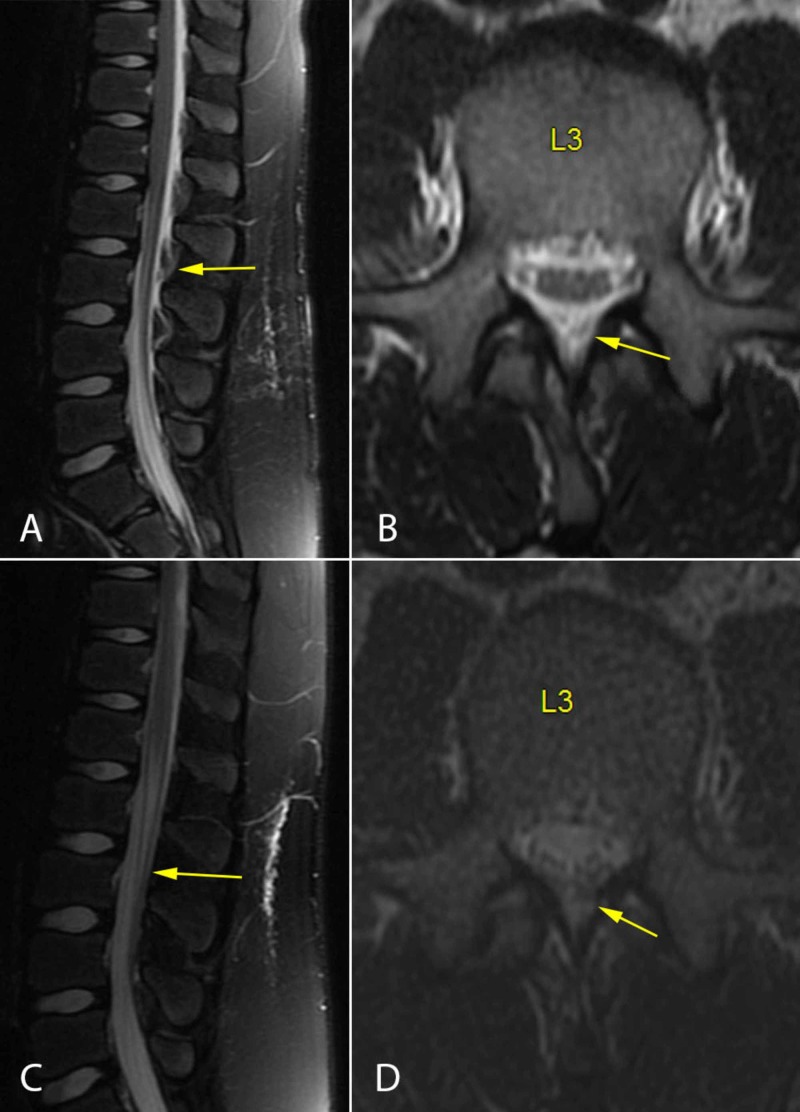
Case 3 Spine MR images showing the compressive epidural fluid collection before treatment (A and B), with resolution after treatment (C and D).
